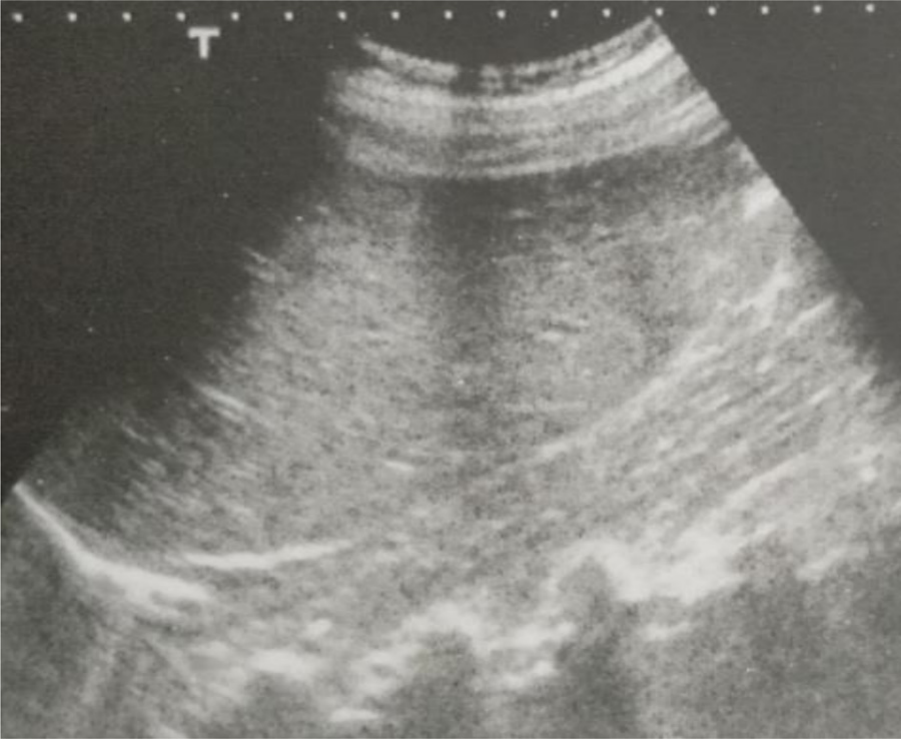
Ultrasound of the abdomen revealed absence of the right kidney.
